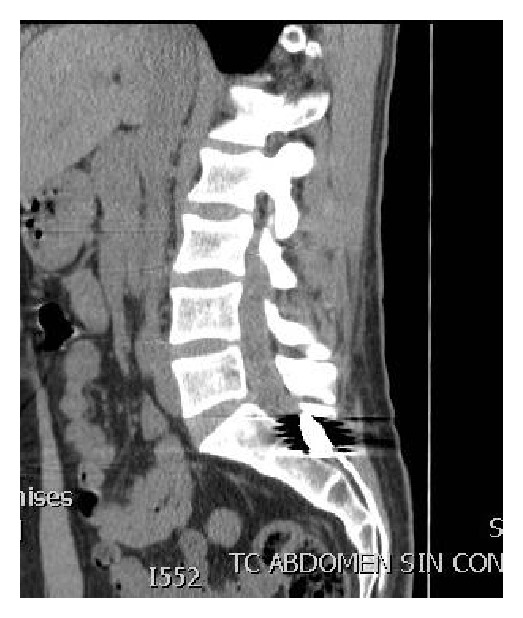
Sagital CT scan shows the bullet lodged at the S2 level.
